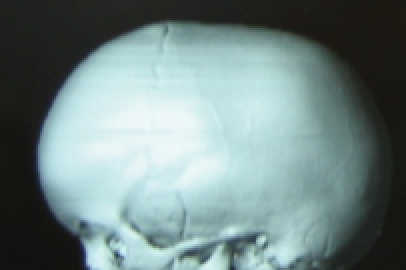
Lateral 3D CT in scaphocephaly
